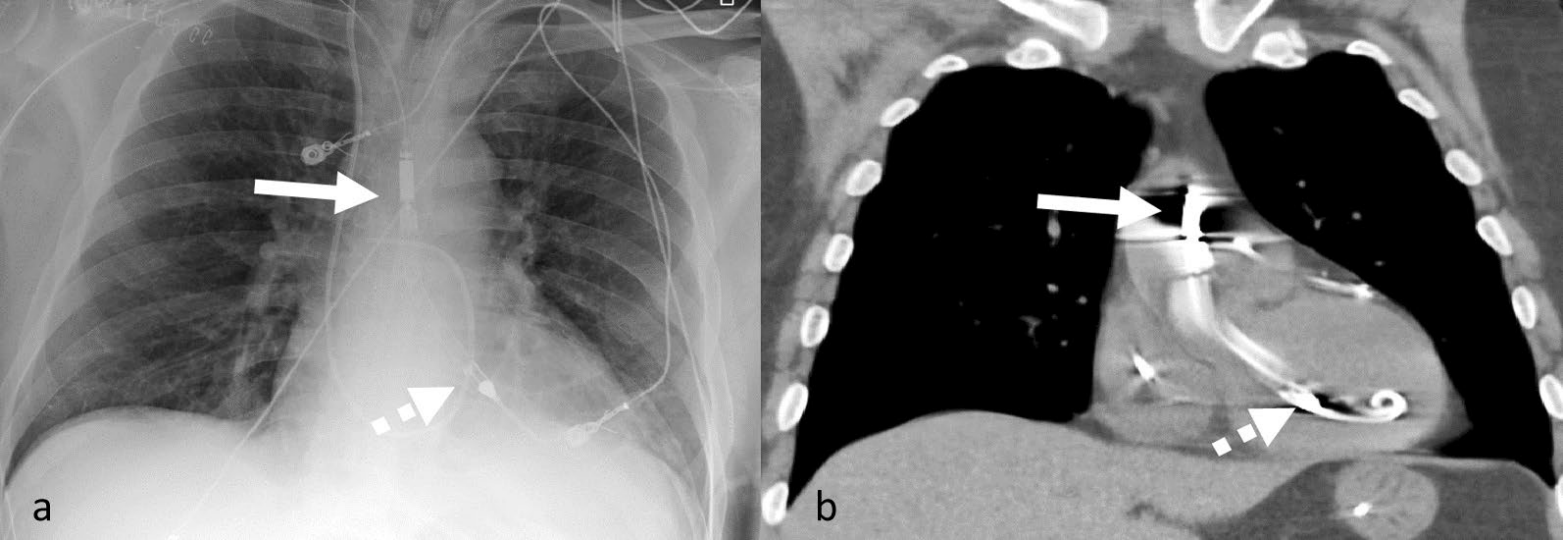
AP chest radiograph (a) and coronal CT (b) demonstrating a correctly positioned transaxillary Impella device. The outlet port is within the ascending aorta (white arrow) and the inlet port is projecting over the left ventricle (dashed arrow). The inlet of Impella CP should be 3.5 cm below the aortic annulus. The outlet should be in the ascending aorta. The pigtail portion is angled toward the LV apex away from the ventricle wall without mitral obstruction. (LV—left ventricle)
